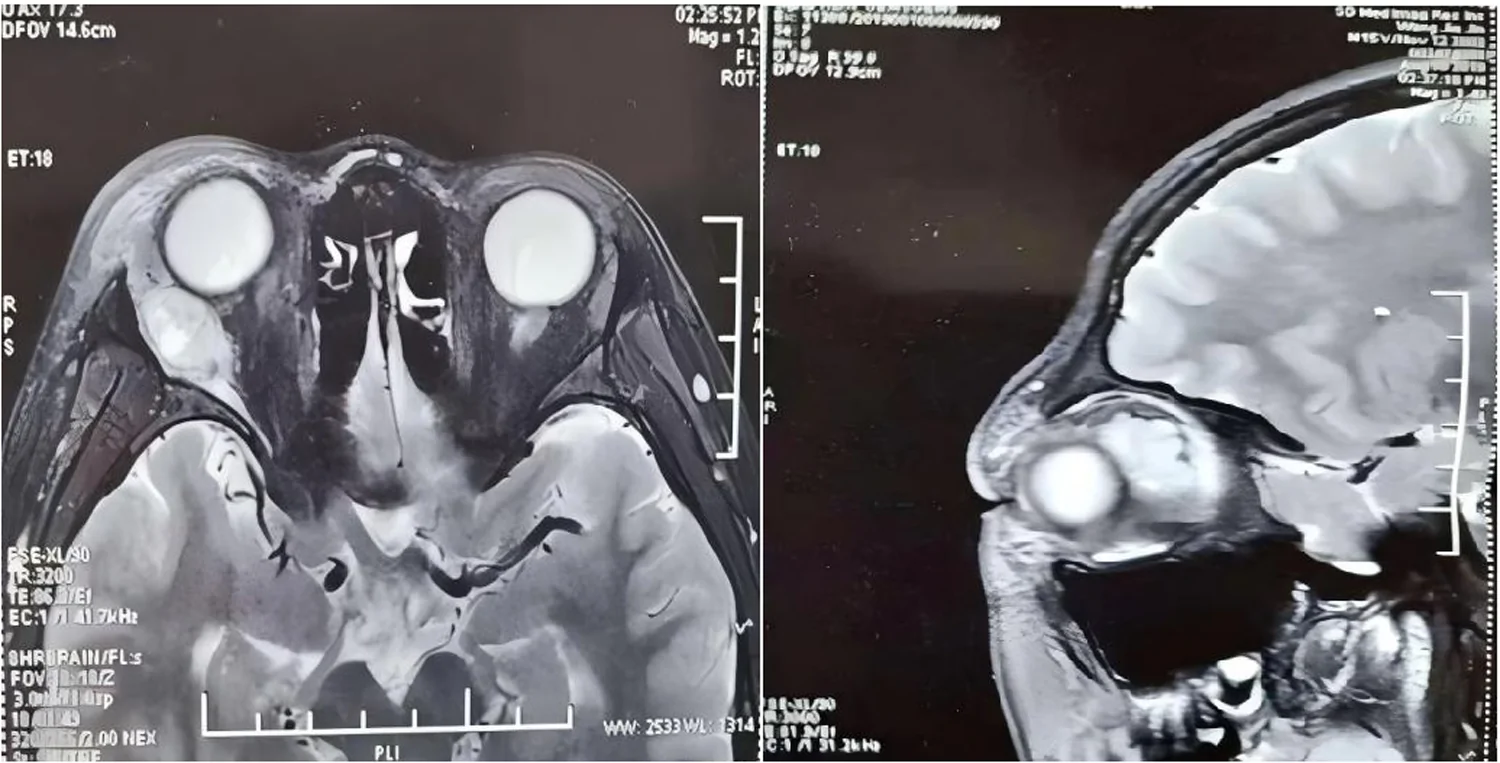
Axial and sagittal fat-saturated T2-weighted orbital MRI shows an extraconal hyperintense soft tissue mass in the right orbit located in the lacrimal fossa and adjacent to the lateral rectus muscle, measuring approximately 2.4 cm × 2.3 cm × 1.4 cm. The left orbital soft tissues appear normal within the imaging field.
